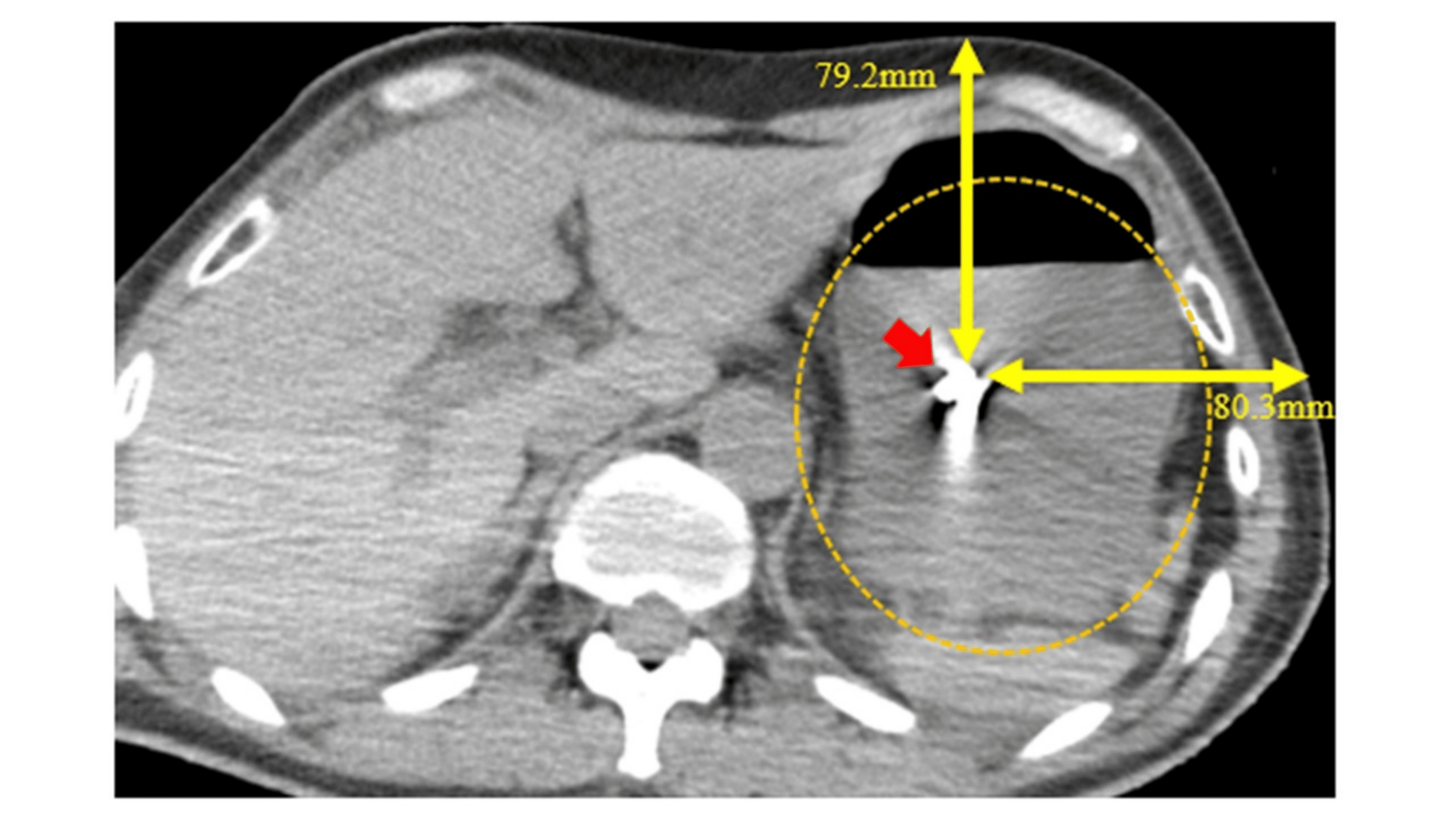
CT image on the day after admission and NGT placement (Case 10) This image shows the NGT tip was away from the abdominal wall (79.2 mm) and buried in the gastric residue. The arrow indicates the NGT tip, the double arrow shows the distance from the NGT tip to the abdominal wall, and the circle marks the gastric residue. CT, computed tomography; NGT, nasogastric tube
